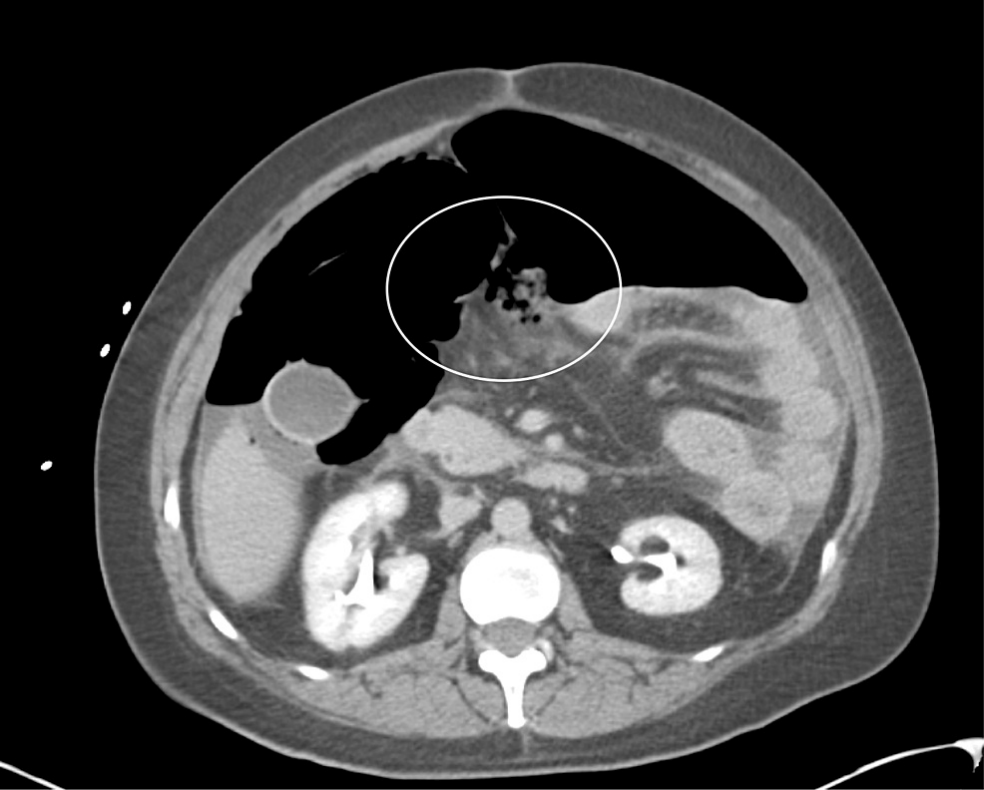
CT abdomen (axial view) showing distention and pneumotosis outside of preparation of small bowel.
